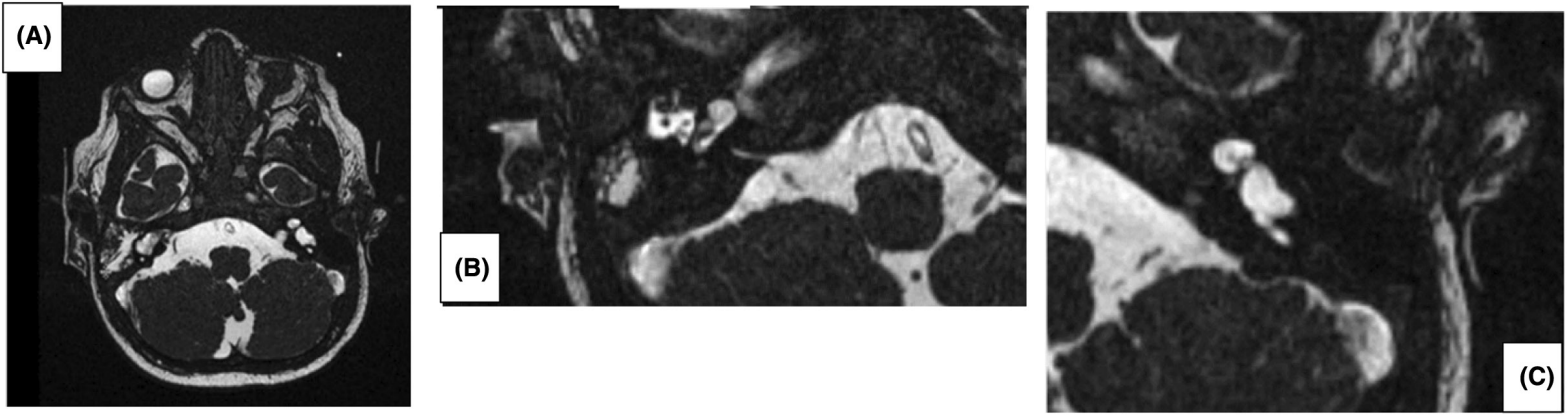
(A) MRI of the inner ear showing a malformation of both inner ears with detailed view of the right (B) and left ear (C). MRI, magnetic resonance imaging.
